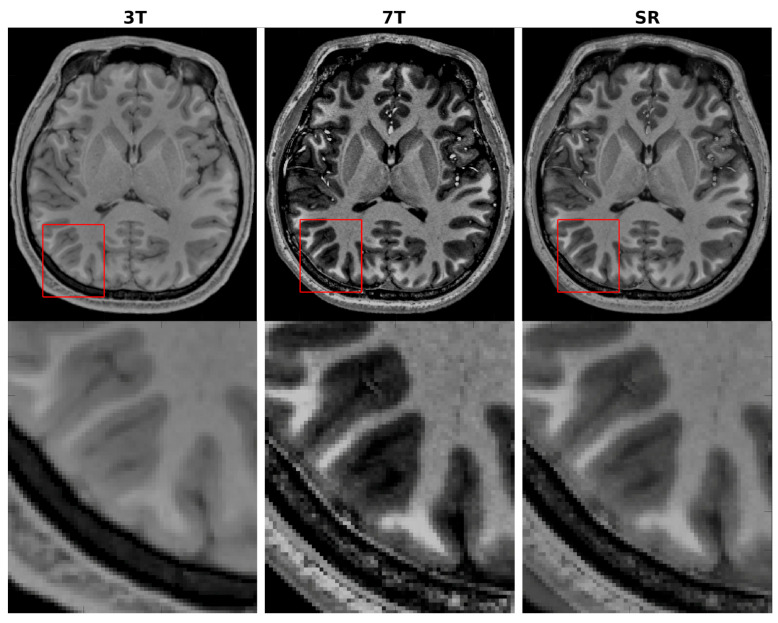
A representative T1w axial slice of the brain as well as a 4-fold zoomed-in display of the selected region marked with a red box showcasing the application of the proposed CHARMS network for SR reconstruction on the 3T MRI data using the paired PTT dataset (PTT2).
